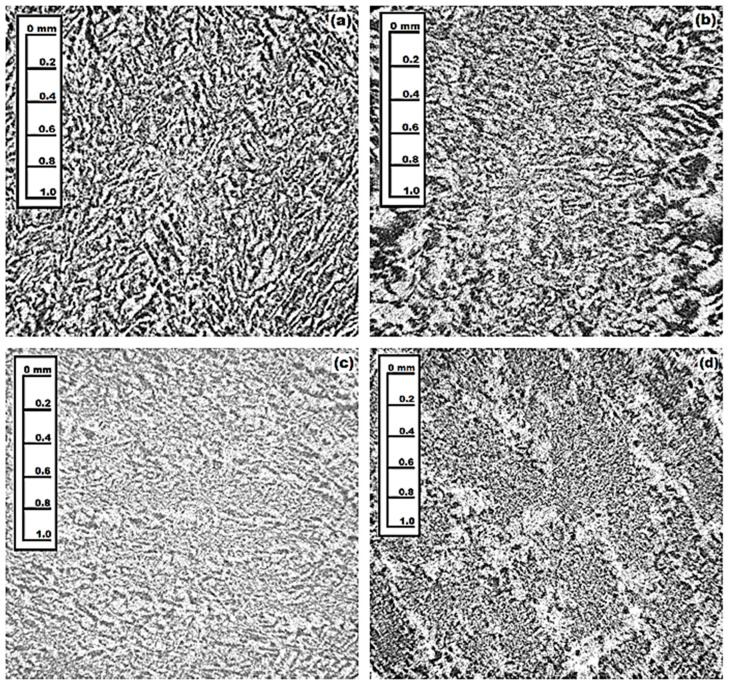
X-ray microtomography of the (Al_2_O_3_-YAG):Ce eutectic: garnet (white) and sapphire (gray) phases distribution for samples 2 (a), 3 (b), 4 (c), and 5 (d).
